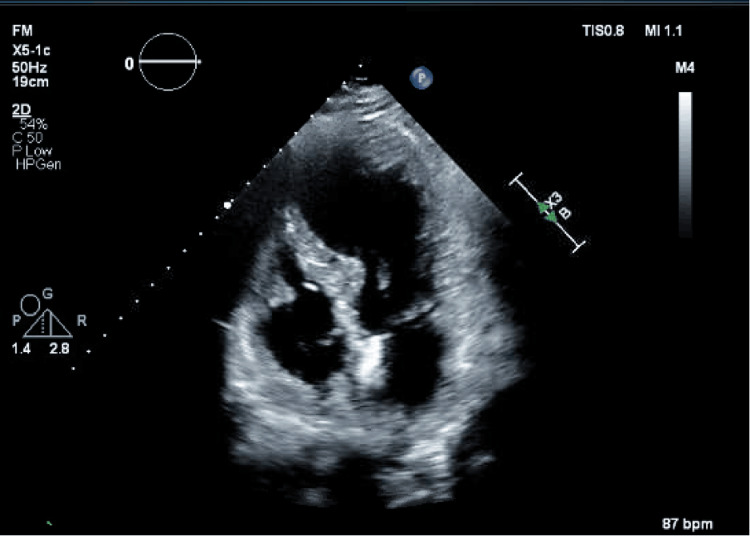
Echocardiogram showing significantly dilated left ventricle with apical ballooning.
